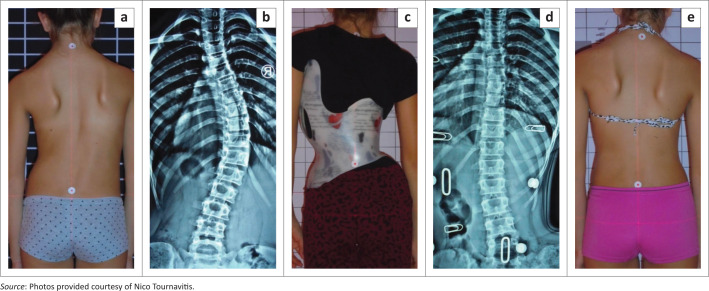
Clinical and radiological intermediate result after 6 weeks. From left to right: (a) initial clinical appearance of a single thoracic scoliosis with (b) 44° of Cobb angle. (c) Patient in brace mirroring of initial deformity is clearly visible. (d) In-brace X-ray with –17° of Cobb angle with a (e) clinical over-correction of the pelvic and the trunk.
